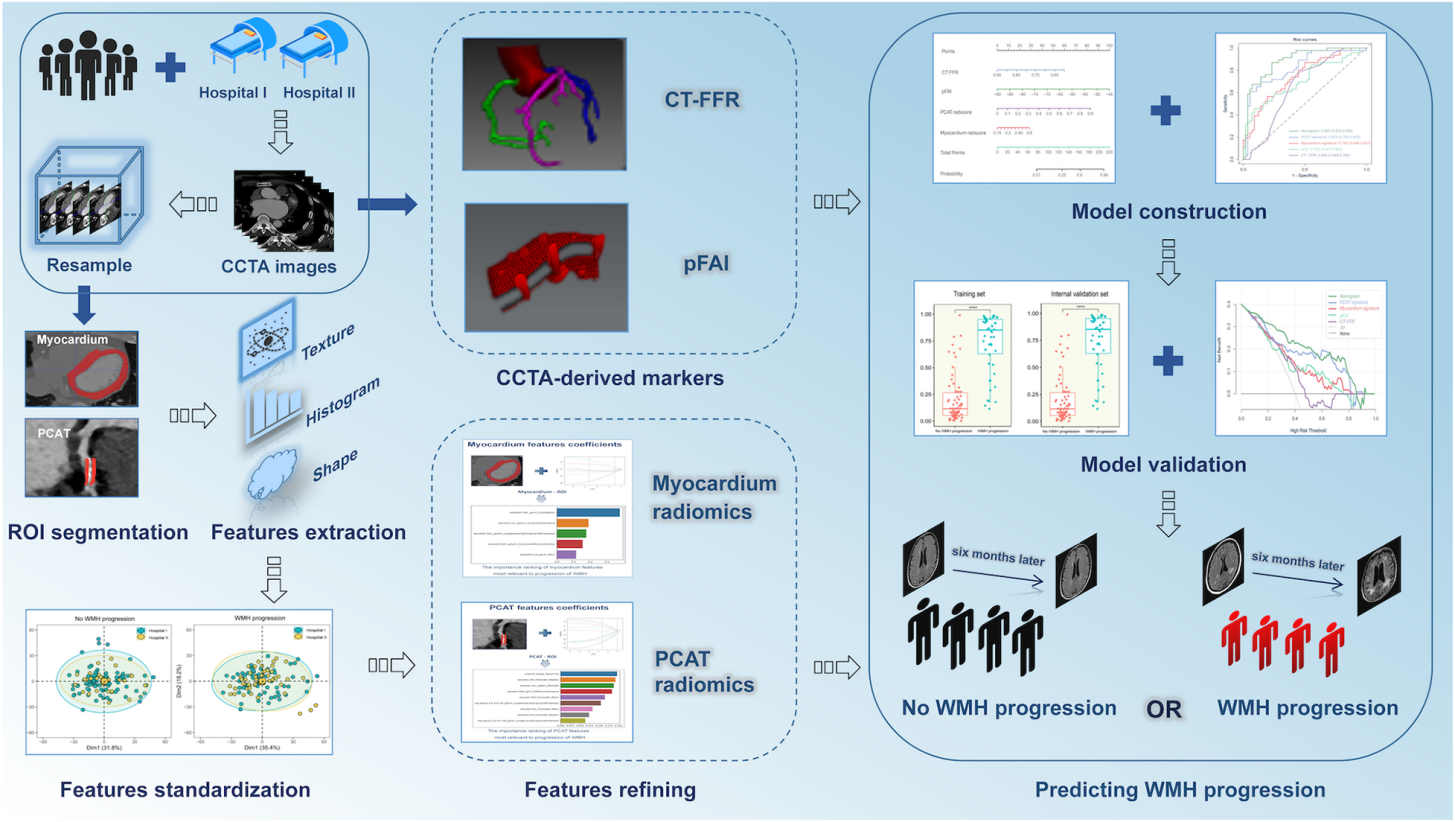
Procedures used for radiomics analysis. CCTA, coronary computed tomography angiography; CT-FFR, CT fractional flow reserve; pFAI, pericoronary fat attenuation index; WMH, white matter hyperintensity.
